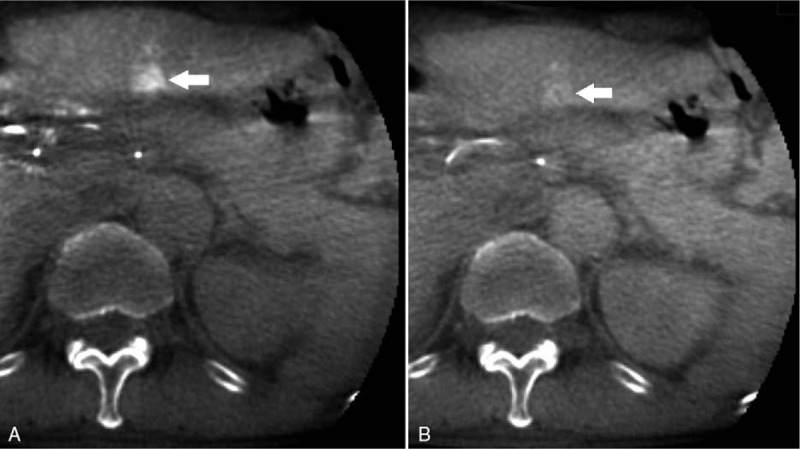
A 70-year-old man with a hypervascular HCC of the segment 3. On the first phase of CBCTHA (A), the nodule was demonstrated as a hypervascular lesion with a diameter of 1.2 cm. The nodule was well defined, but has an irregular shape. Therefore, all readers rated the score of the nodule as 2 with a low confidence level. On the second phase of CBCTHA (B), corona enhancement was well demonstrated. The rating score for dual-phase CBCTHA imaging was up to 3 in all readers with a high confidence level.
